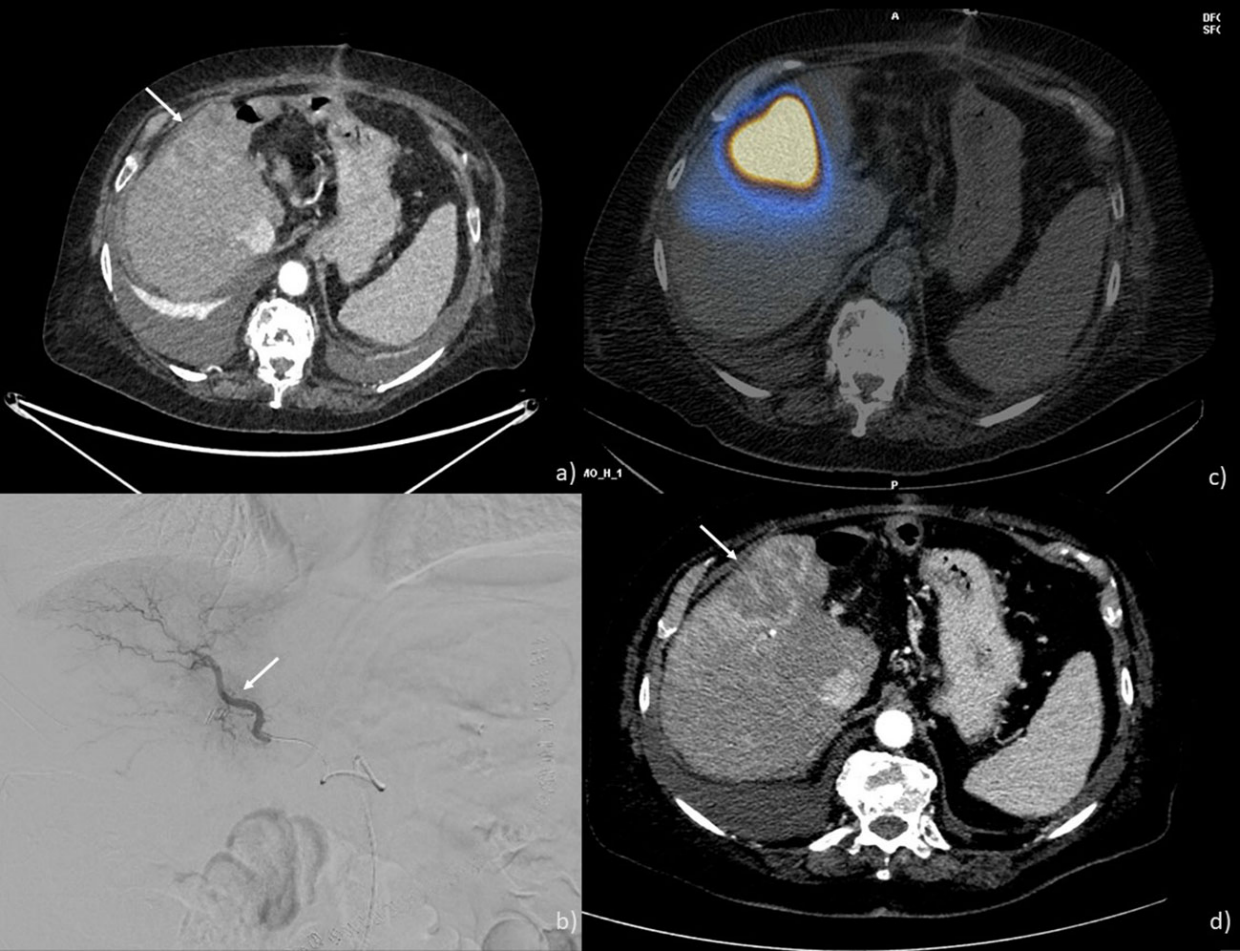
TARE performed on a 69-year-old man: (A) CT scan showing an ICC nodule in the 5th and 8th hepatic segments (arrow); (B) angiographic study showing ICC nodule the placement of the microcatheter at the level of the branch for the anterior segments (arrow); (C) SPECT-CT after Tc-99m macro aggregated albumin injection showing a good coverage of the lesion (D) CT scan post-treatment showing a good response with necrosis of the lesion (arrow).
